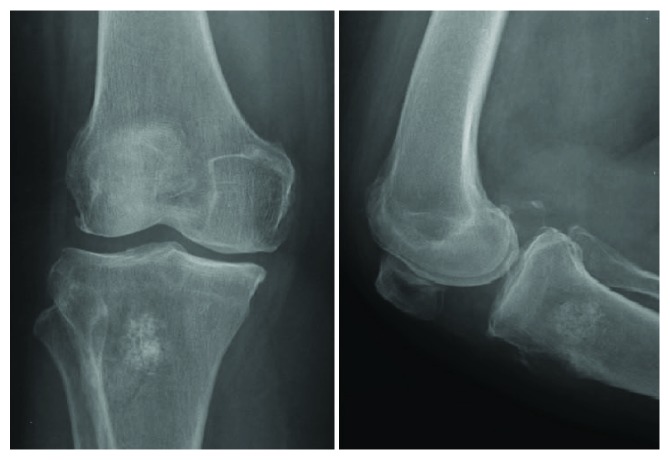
X-ray of the right knee reveals a mixed sclerotic and lytic lesion in the proximal aspect of the tibia with anterior cortical disruption.
